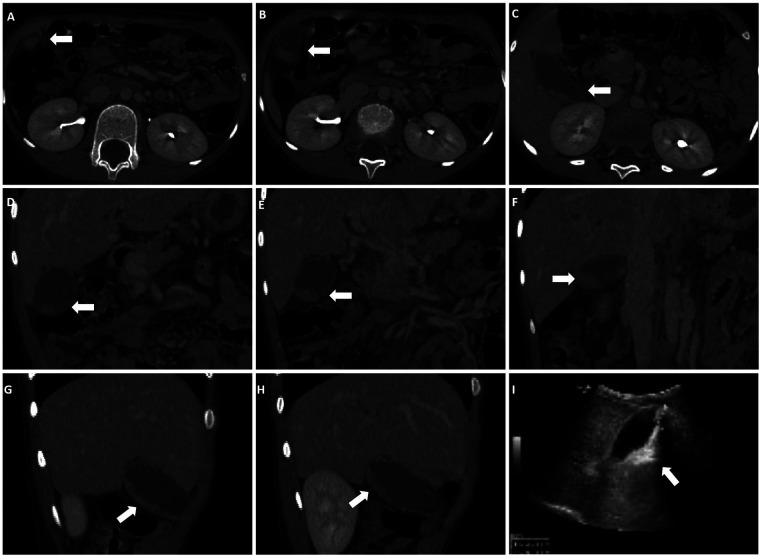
Both CT and ultrasound examinations reveal PL. (A–C) Cross-sectional CT images. (D–F) Coronal CT images. (G,H) Sagittal CT images. (I) ultrasound shows a 3.1 × 1.0 cm strong echo within the gallbladder lumen, accompanied by acoustic shadowing. Arrows indicate the presence of high-density shadows and strong echoes within the gallbladder.
